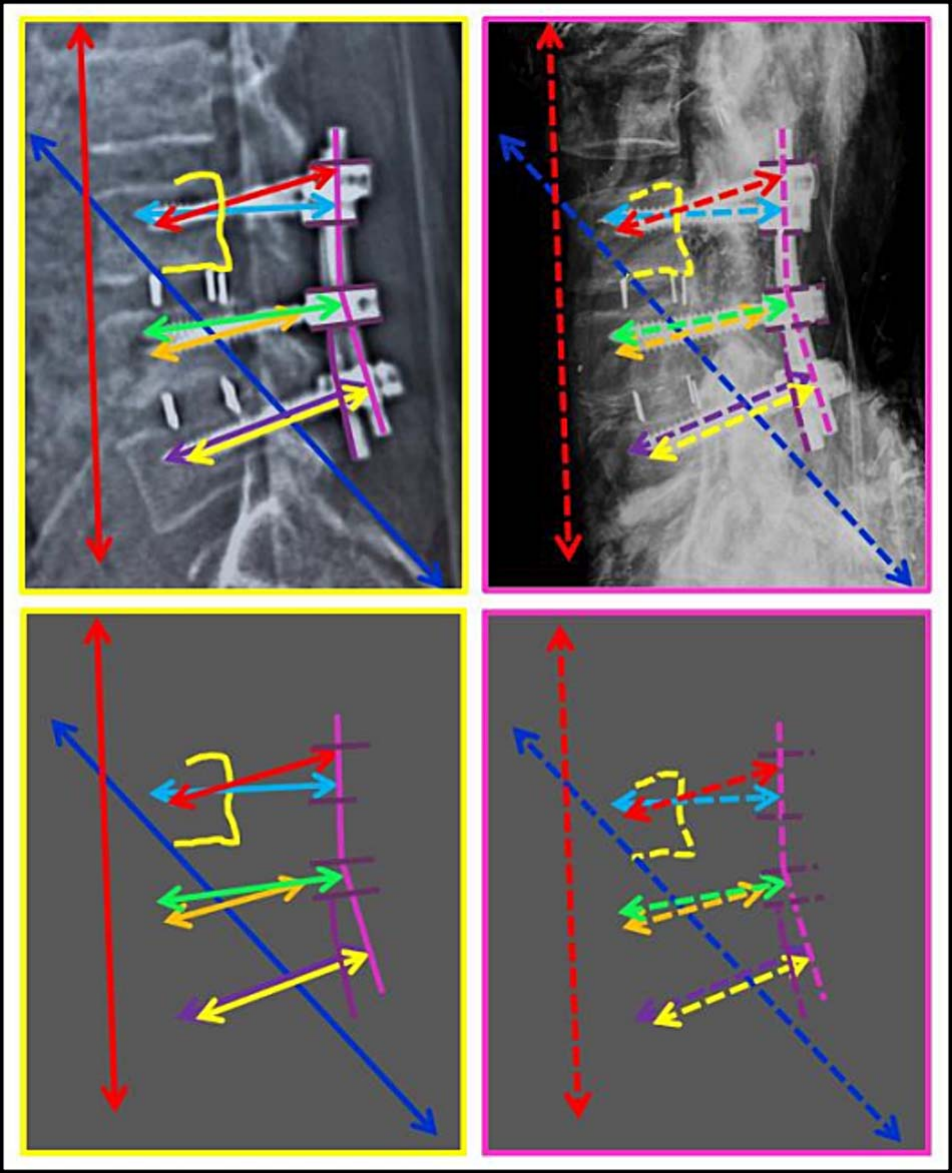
Proportion and positioning of the interposition of lines interconnecting specific anatomical points about the rods and screws. Upper panels, comparison between the antemortem CT images (left) and postmortem lateral X-ray (right). Lower panel, respective concordant templates.
